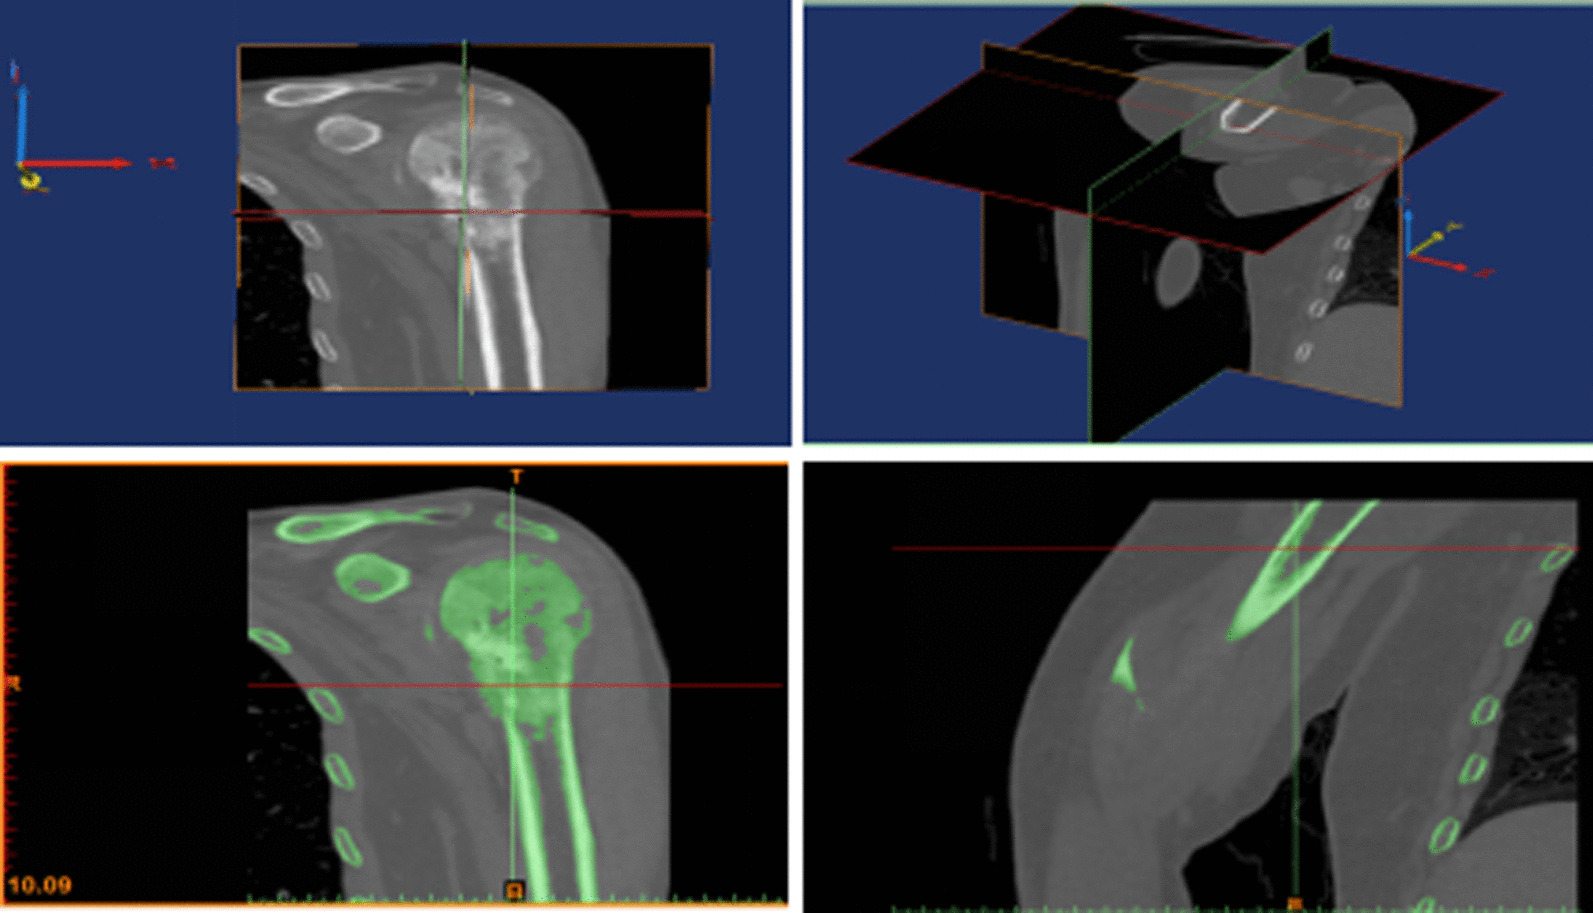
Preoperative design of primary malignancies and metastases Preoperative DICOM data from CT scan of humerus were transferred to Mimics software system (Fig. 2). The range of bone damage within the lesion was drawn by threshold segmentation. The maximum diameter of the tumor was measured by combining coronal, sagittal, and transverse data, and the location of array and the number of thermal fields were estimated, and the overlap of each potential hyperthermia range was noted
